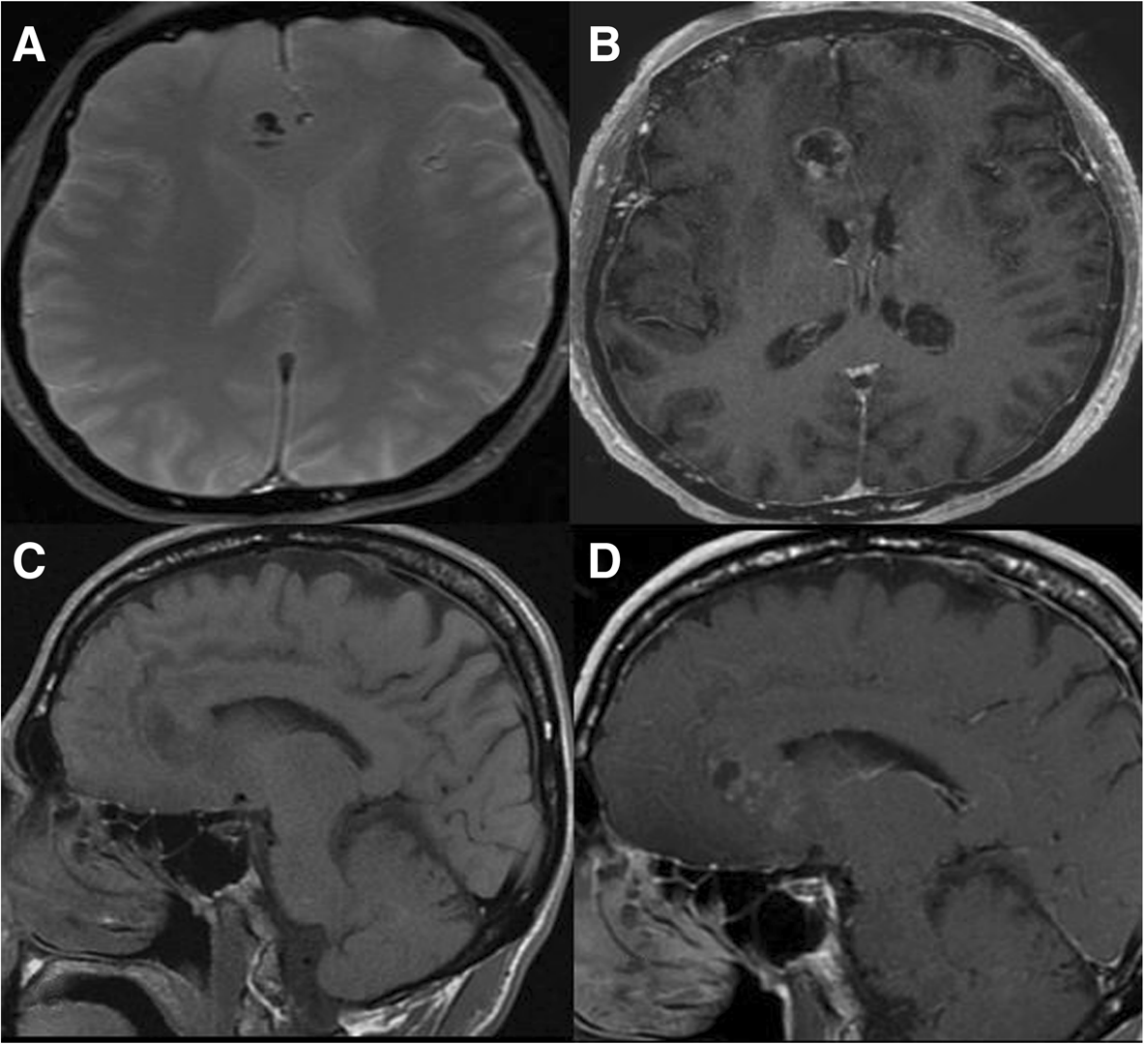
Axial echo gradient (A) and T1 postcontrast (B) and sagittal precontrast (C) and postcontrast (D) magnetic resonance imaging scans showing peripheral contrast uptake and a hemorrhagic heterogeneous lesion located in the right cingulate gyrus.
